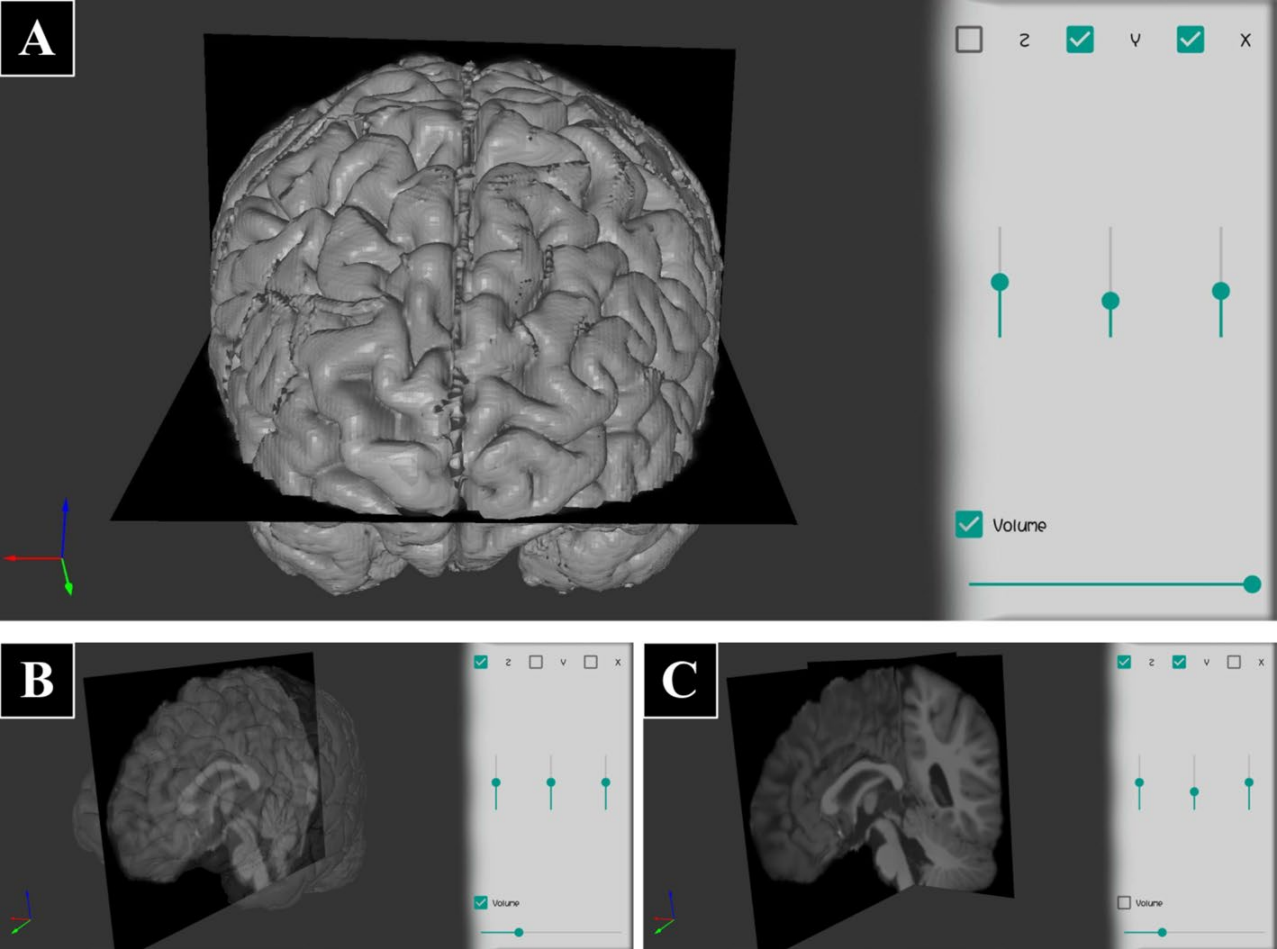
A brain MRI volume (without skull) displayed with different visualization options (Dataset IV). A Two slices, in axes “*X*” and “*Y*” of the volume, and a volume rendering of the image with maximum alpha, visualizing the cortical surface. B Only “*Z*” axis slice, and the volume rendering with alpha = 0.3 (semi-transparent). C Only two slices displayed, in axes “*Y*” and “*Z*”
